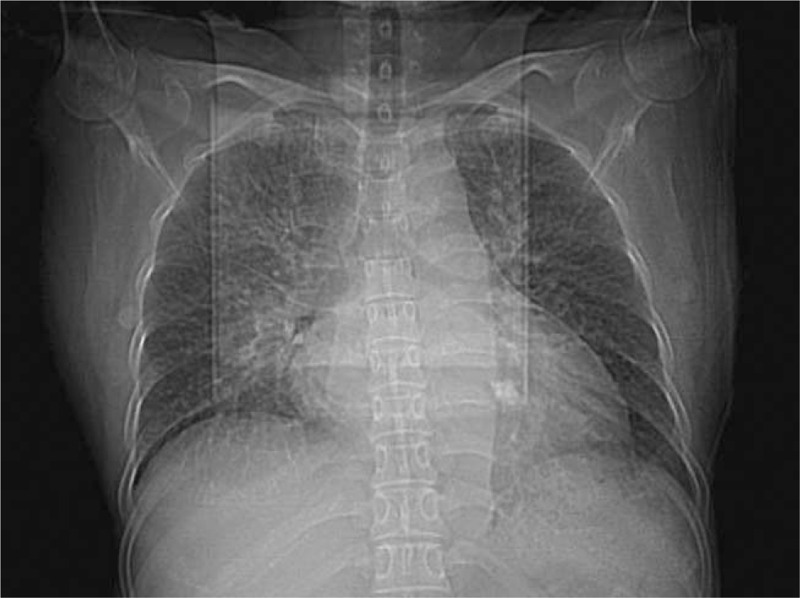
Chest radiograph: Cardiothoracic ratio of 0.69.
